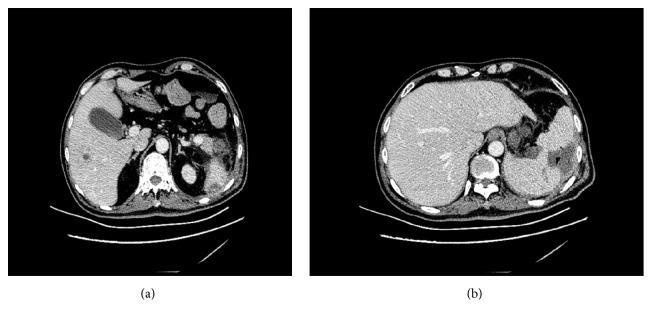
A contrast-enhanced CT scan of abdomen showed (a) rounded hypodense lesion with central low attenuation in the right lobe of the liver (suggestive of liver abscess) and (b) hypodense splenic air-filled lesion with an irregular wall (suggestive of perisplenic infection).
